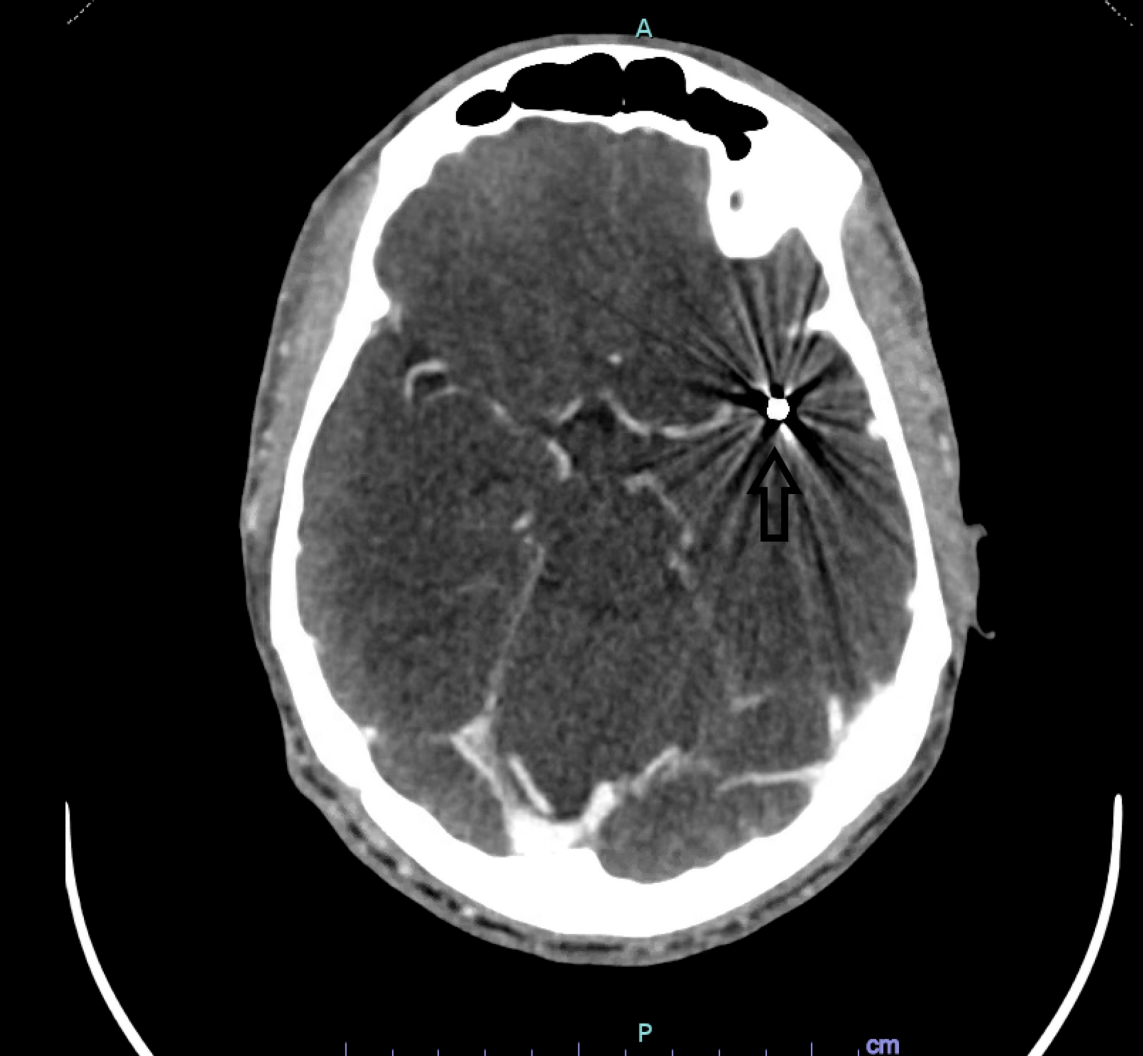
CTA head demonstrating pellet in left MCA distal M1 or proximal M2 branch (streak artifact limits exact localization)
